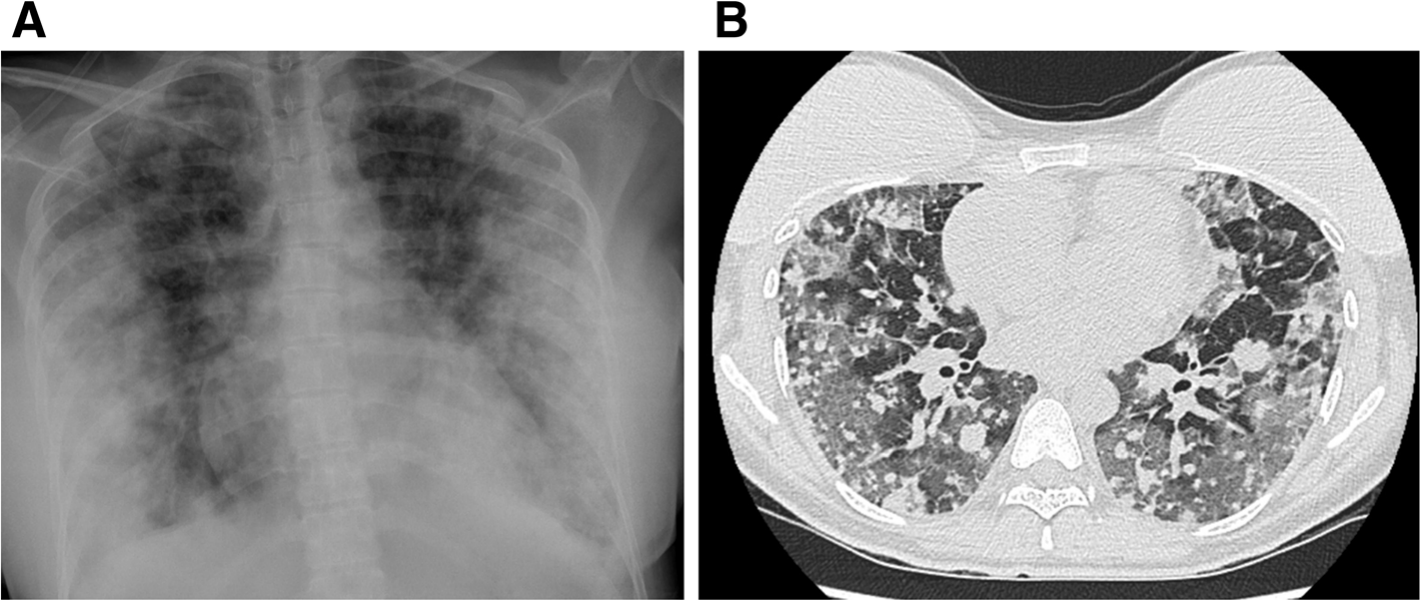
a Scout Image from Chest CT, revealing diffuse ground-glass opacification and pulmonary infiltrates. b Contrast-enhanced chest CT, presenting signs of diffuse alveolar hemorrhage and multiple pulmonary nodules
